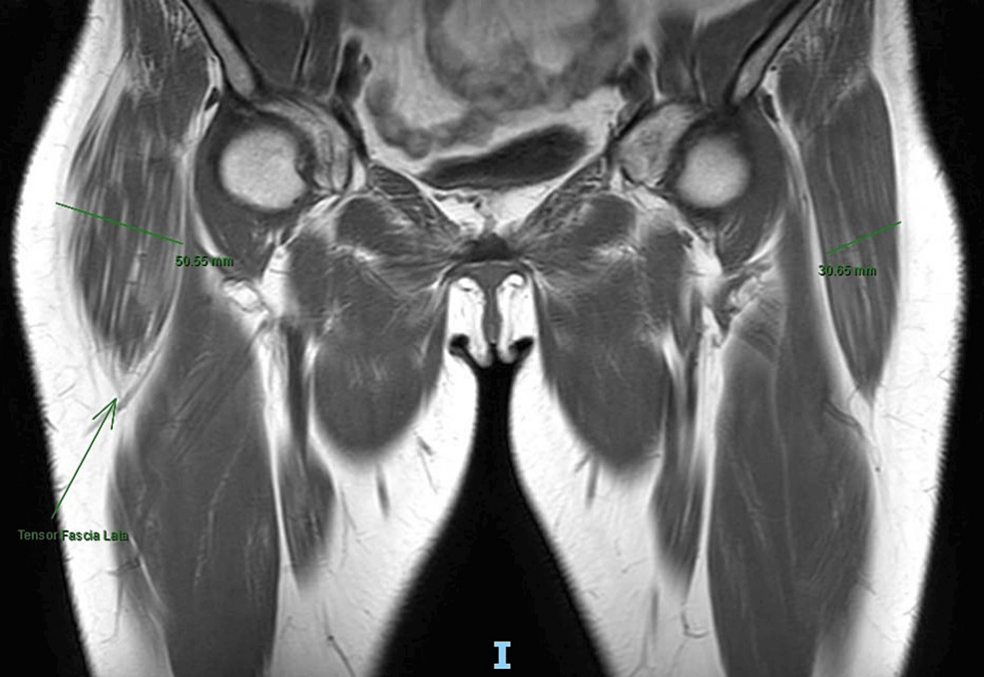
T1-Weighted MRI: Coronal cut Hypertrophied TFL (arrow) and both TFL measurements (50.55 mm Right and 30.65 mm Left). TFL: tensor fasciae latae
